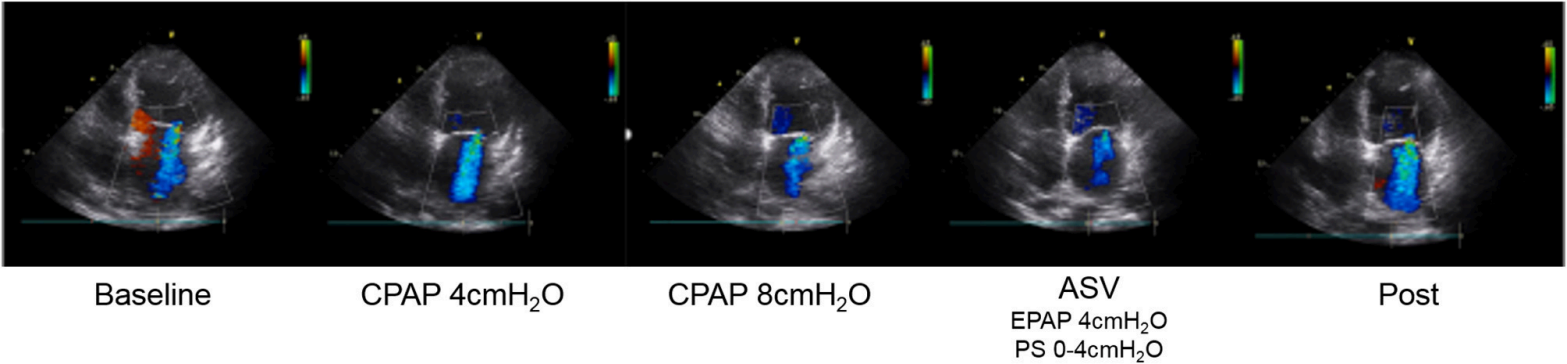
Representative color Doppler images of functional MR. MR jet area fraction reduced due to PAP therapy, especially CPAP at 8 cm H_2_O and ASV. ASV, adaptive-servo ventilation; CPAP, continuous positive airway pressure; EPAP, expiratory positive airway pressure; MR, mitral regurgitation; PAP, positive airway pressure; PS, pressure support.
